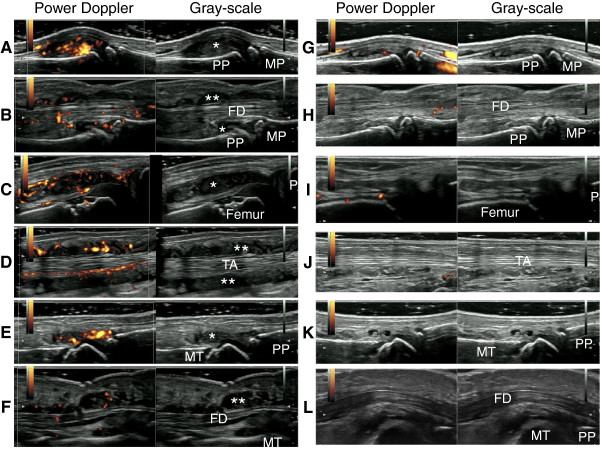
Representative ultrasound images of intra-articular synovitis and tenosynovitis in patient 5. Shown are the representative ultrasound images of intra-articular synovitis (A, C, E, G, I, K) and tenosynovitis (B, D, F, H, J, L) in patient 5 before (A-F) and after treatment (G-L). All images before treatment demonstrate synovial/tenosynovial hypertrophy, which accompanies increased power Doppler signals (A-F). Additional movie files show this in more detail (see Additional files
